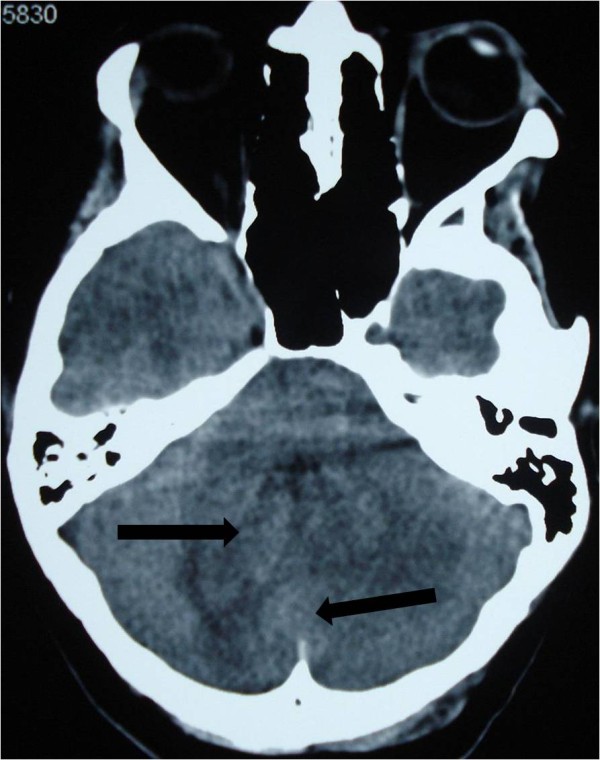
Cerebral computed tomography scan of two lesions in the right cerebellar hemisphere and the vermis (arrow). The lesions are spontaneously hypodense.
